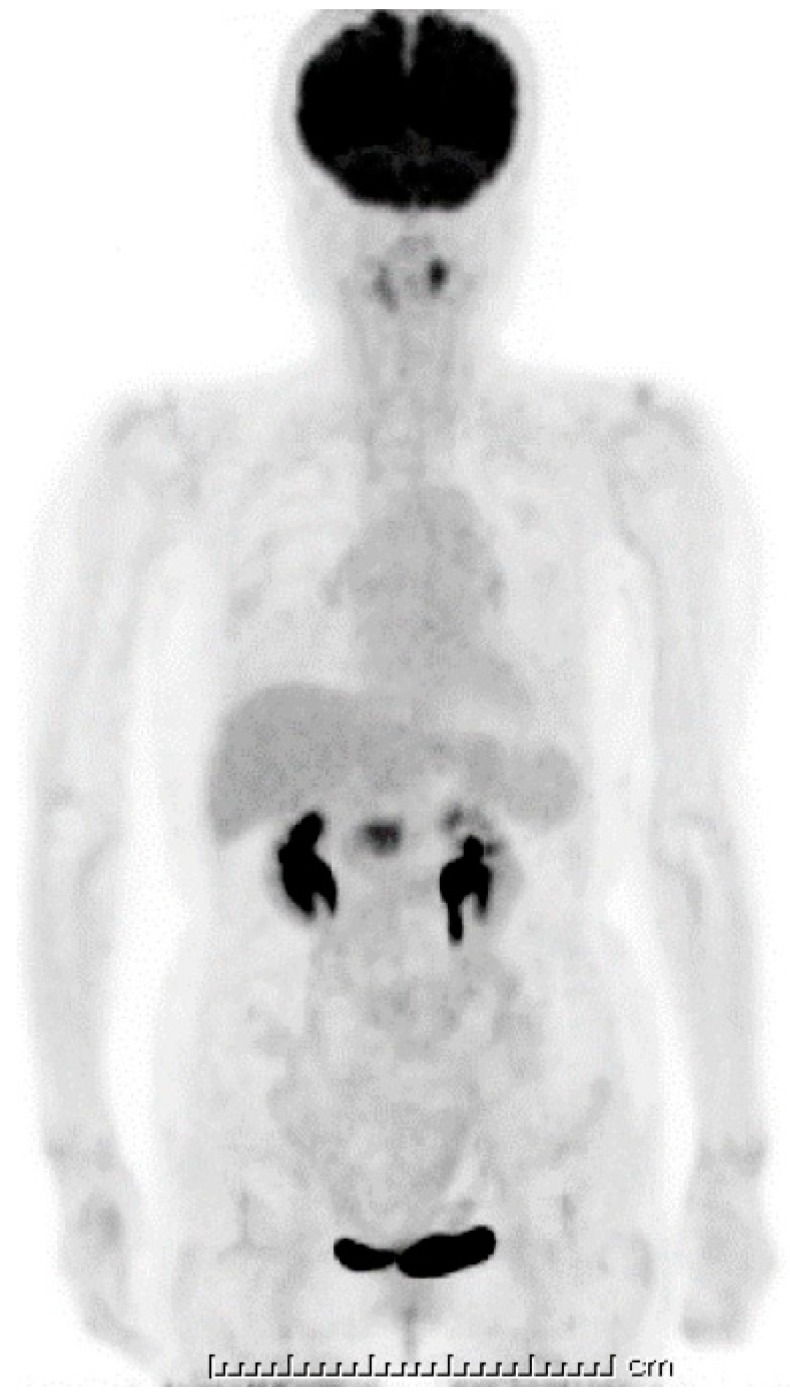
^18^F-fluorodeoxyglucose positron emission tomography (FDG-PET). A strong uptake of FDG was visible at the pancreas head (SUVmax: 6.95); however, this looked smaller when compared with the initial computed tomography (CT) image. The one unit of under bar is indicating 1cm and total bar length is 25 cm.
